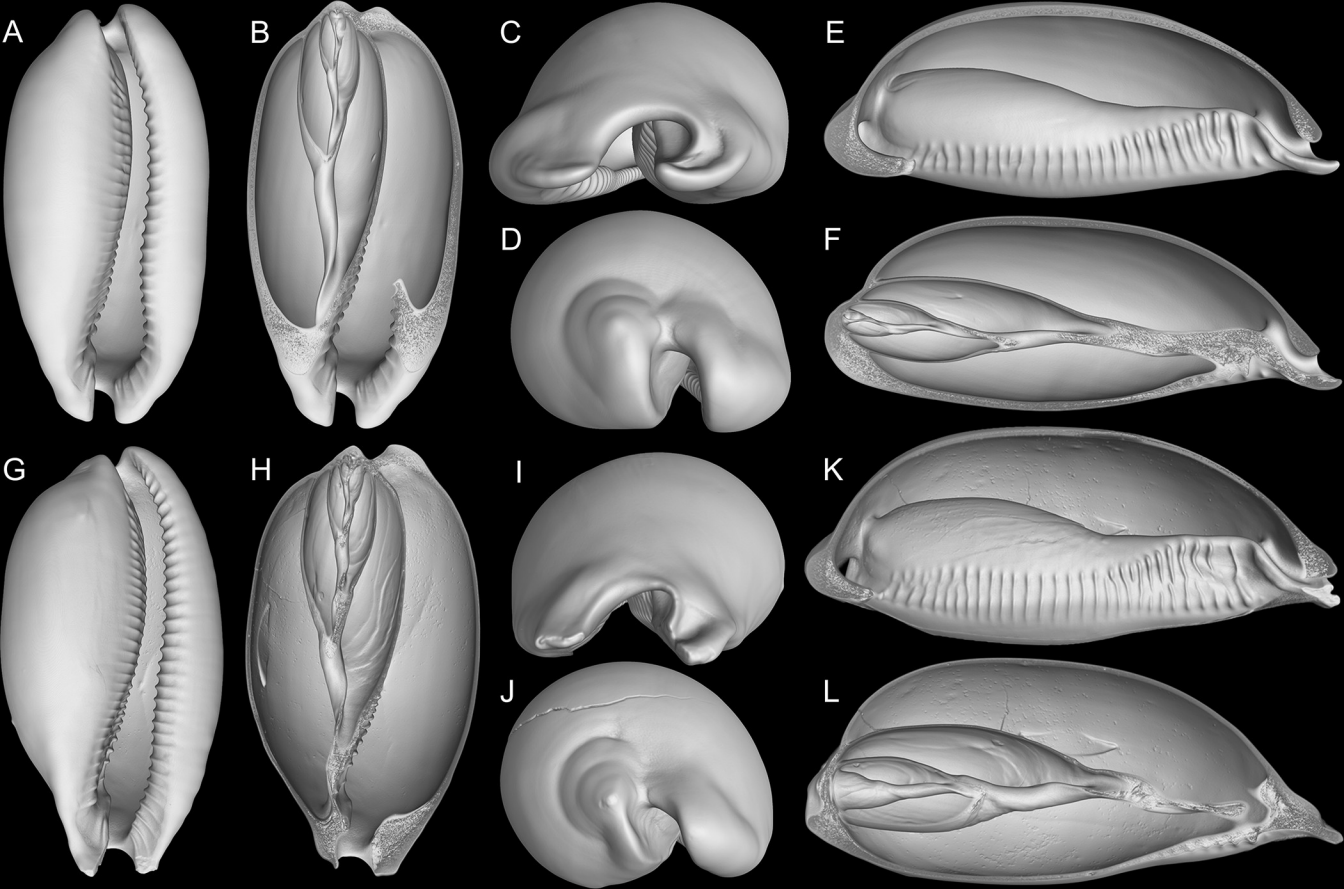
Macrocypraea*, 3D reconstructions of shells (CT-Scan). (A-F) *M*. *cervinetta* MZSP 131503 (L 58 mm). (A) Frontal view. (B) Same, more ventral portions removed. (C) Anterior view; (D) Posterior view. (E) Right view, right half removed. (F) Same, longitudinal section along columellar axis. (G-L) *M*. *zebra* MZSP 38946 (L 100 mm). (G) Frontal view. (H) Same, more ventral portions removed. (I) Anterior view. (J) Posterior view. (K), Right view, right half removed. (L) Same, longitudinal section along columellar axis.
